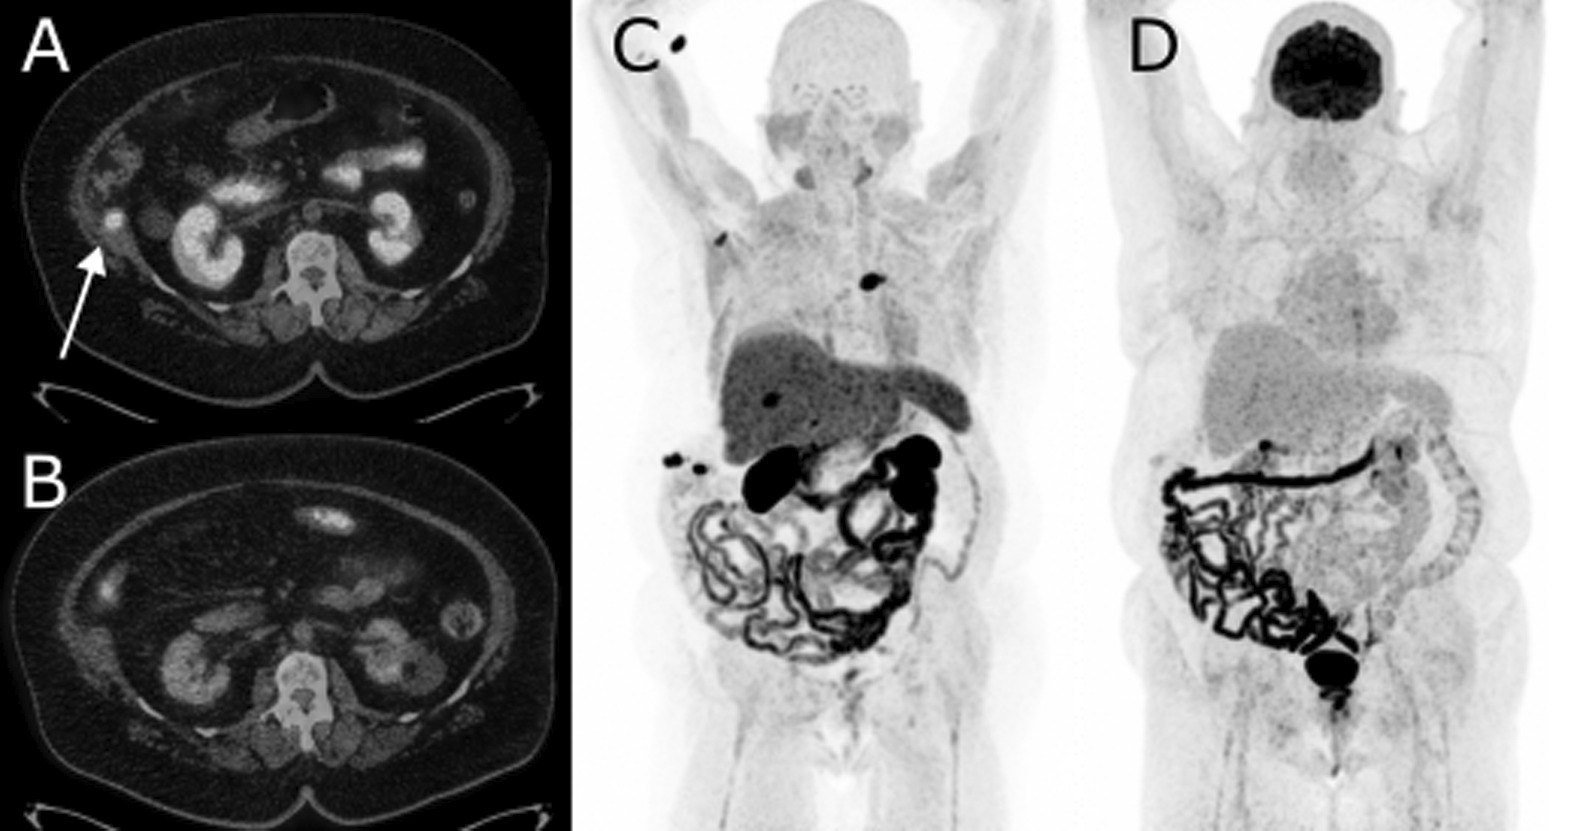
This 75-year-old female patient (#8) with HCC in segment VI and VII, both successfully treated with MWA in 2018 and 2020. Post-treatment MRI follow-up at three months revealed two new lesions in segment V and VIII and several abdominal wall lesions suspected of needle tract metastasis. Dual-tracer PET/CT showed increased uptake of [11C]CH (A) for a needle tract site metastasis near the 10th rib (arrow), not seen by [18F]FDG (B). In addition, maximum intensity projection (MIP) of [11C]CH (C) revealed several intrahepatic metastases and a lesion at fifth costovertebral junction, all not seen on [18F]FDG (D). The costovertebral lesion resulted rapidly into spinal cord injury to which patient received emergency radiotherapy. Patient received palliative pain care and died shortly after
